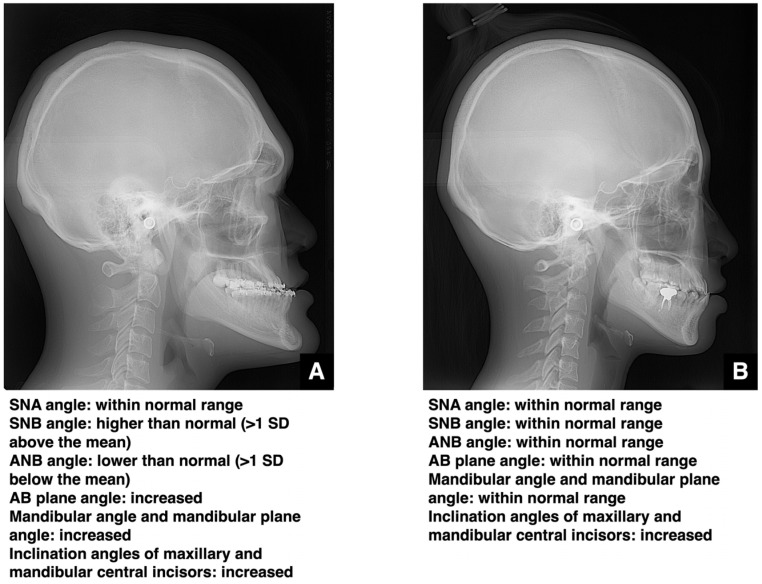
Analysis of skeletal and dentoalveolar mandibular prognathism. (A) Lateral cephalometric radiograph and summary of polygonal analysis of a patient with skeletal mandibular prognathism. (B) Lateral cephalometric radiograph and summary of polygonal analysis of a patient with dentoalveolar mandibular prognathism. Permission has been obtained to publish all figures.
